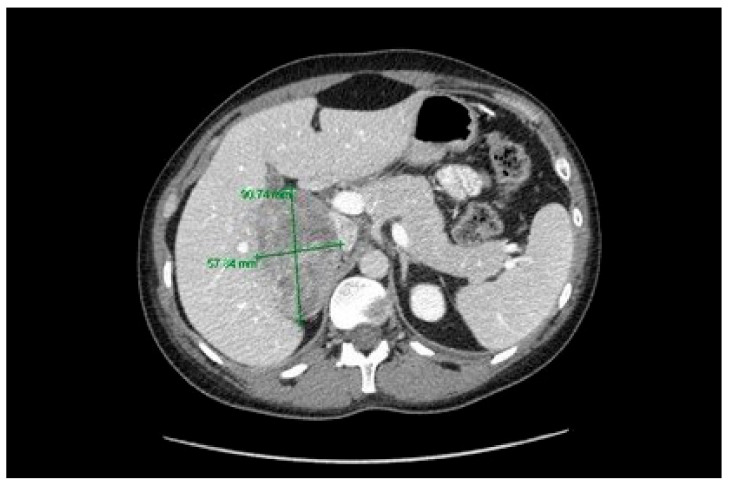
Computed tomography revealing the 9.1 × 5.8 cm right adrenocortical mass.
